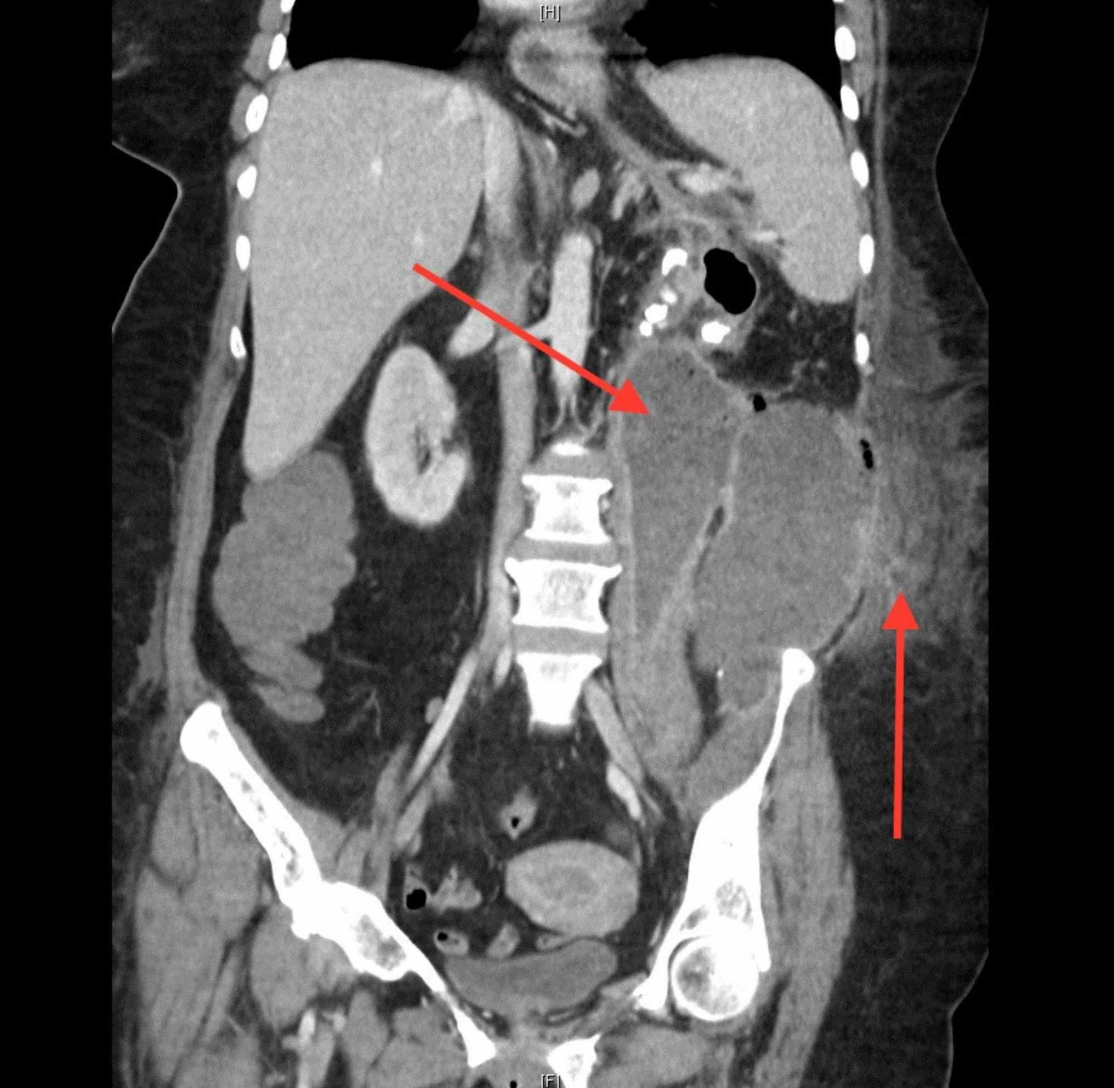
Contrast-infused CT: Subcutaneous extension of abscess with septation The left arrow re-identifies the enlarging, and now septated, perinephric abscess with extension into the pelvic cavity. The right arrow identifies infiltration of abscess into the subcutaneous tissue external to the peritoneal cavity.
